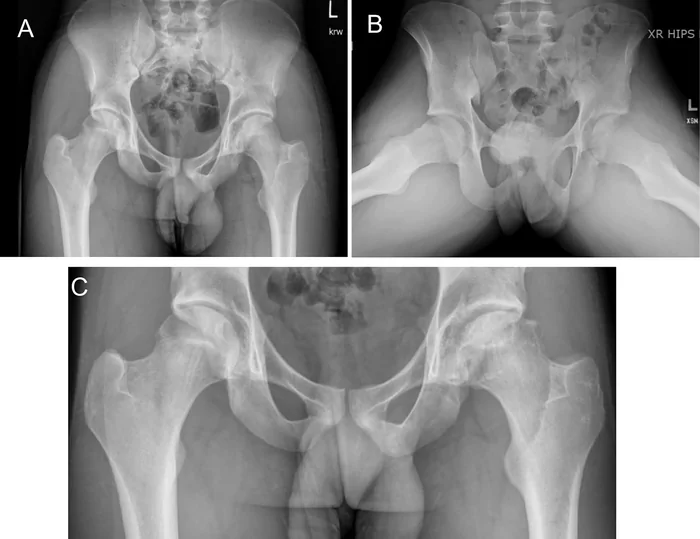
(A) Bilateral pelvic anterior-posterior radiograph 6 weeks postoperatively shows intact left hip osteochondral allograft with maintained joint space. (B) Bilateral frog lateral radiograph 3 months postoperatively shows excellent position of the left femoral head osteochondral allograft with no signs of collapse. (C) Bilateral pelvic anterior-posterior radiograph 24 months postoperatively shows minimal sclerosis in the left hip with small lateral femoral head bone spur formation, maintained left hip joint space, and intact left hip osteochondral allograft. Continued but diminished lucency is seen at the base of the left femoral head osteochondral allograft graft.
